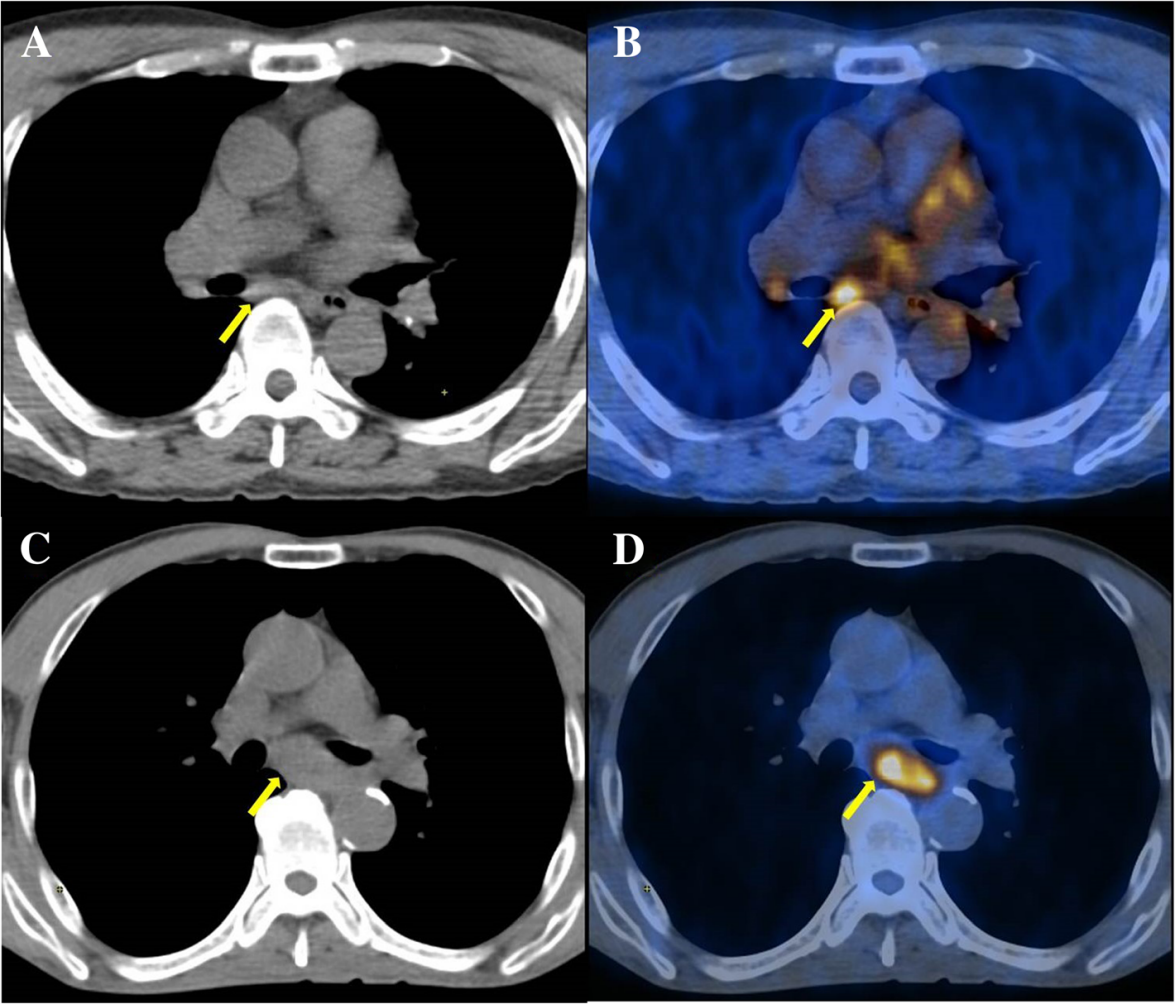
PET/CT image of a 64-year-old male with oesophageal cancer showed lymph node enlargement in subcarinal area. The lymph node’s CT attenuation was high as 112 HU (a) with SUV_max_ of 4.4 (b), and this lesion was revealed to be benign lymph node after surgery. Meanwhile, PET/CT image of a 65-year-old male oesophageal cancer patient with lymph node enlargement in subcarinal area showed a low CT attenuation of 23 HU (c) with a SUV_max_ of 4.4 (d) which revealed to be a metastatic lymph node. *CT* computed tomography, *SUV*_*max*_ Maximum standardized uptake value
